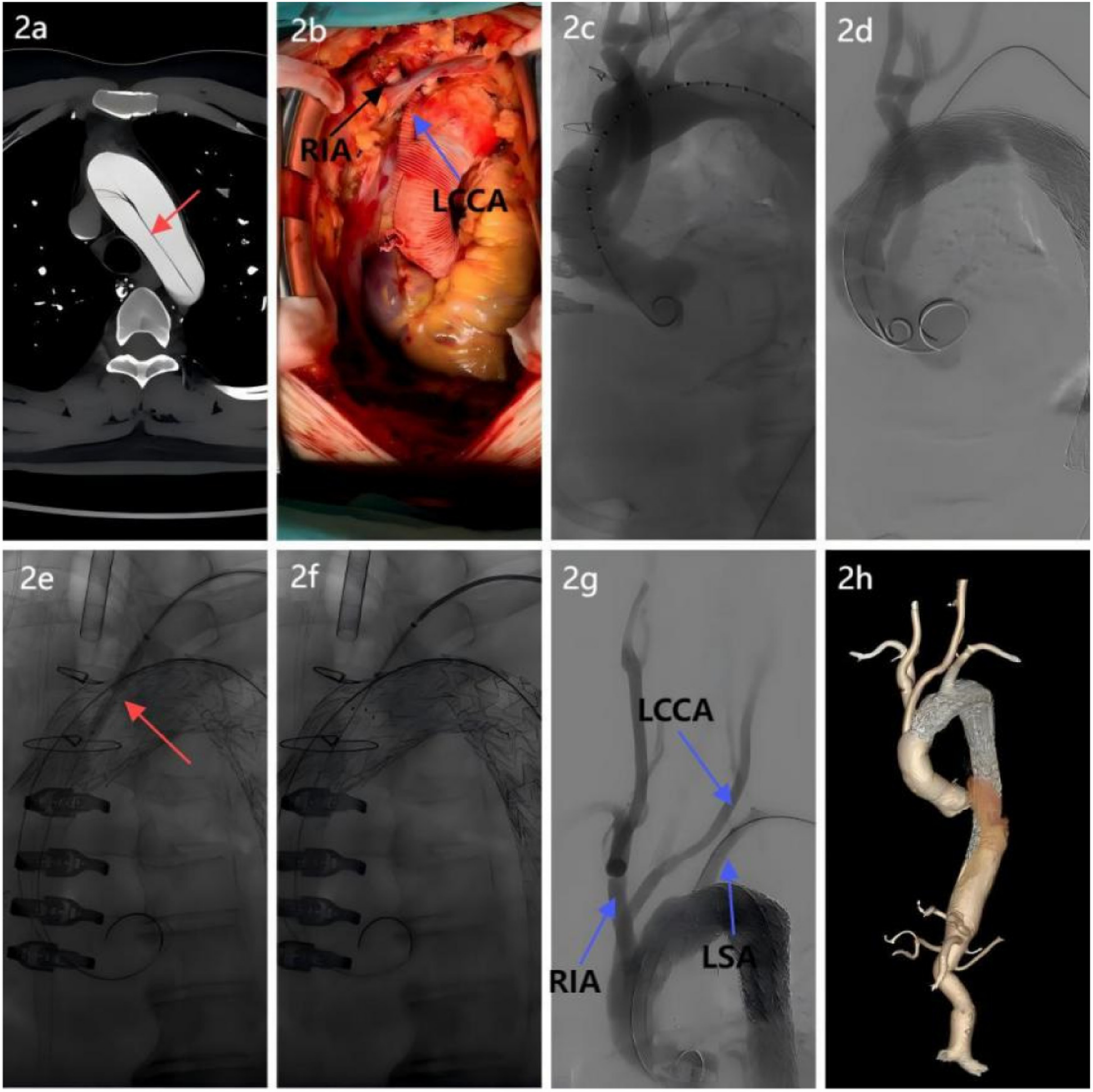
Surgical procedure breakdown. (a) Aortic CTA revealed an aortic arch dissection, necessitating reconstruction of the arch branch vessels; (b) A four-branched prosthetic graft was used: proximal and distal ends were anastomosed end-to-end with the ascending aorta. Two branches were anastomosed to the right innominate artery and left common carotid artery to restore cerebral perfusion; (c) Intraoperative angiography verification confirmed patency of the prosthetic graft and branches, with no anastomotic leaks; (d) A covered stent was implanted distal to the four-branched graft to exclude the false lumen and seal the dissection; (e) After laser fenestration of the left subclavian artery, a balloon was introduced, and balloon dilation revealed a significant notch; (f) A “parachute” technique was used to ensure proper stent apposition; (g) Postprocedural aortography confirmed the patency of all three supra-aortic branches with no evidence of endoleak; (h) Follow-up postoperative CTA of the entire aorta demonstrated patency of the three arch branch vessels, satisfactory stent morphology and position, and absence of endoleak.
